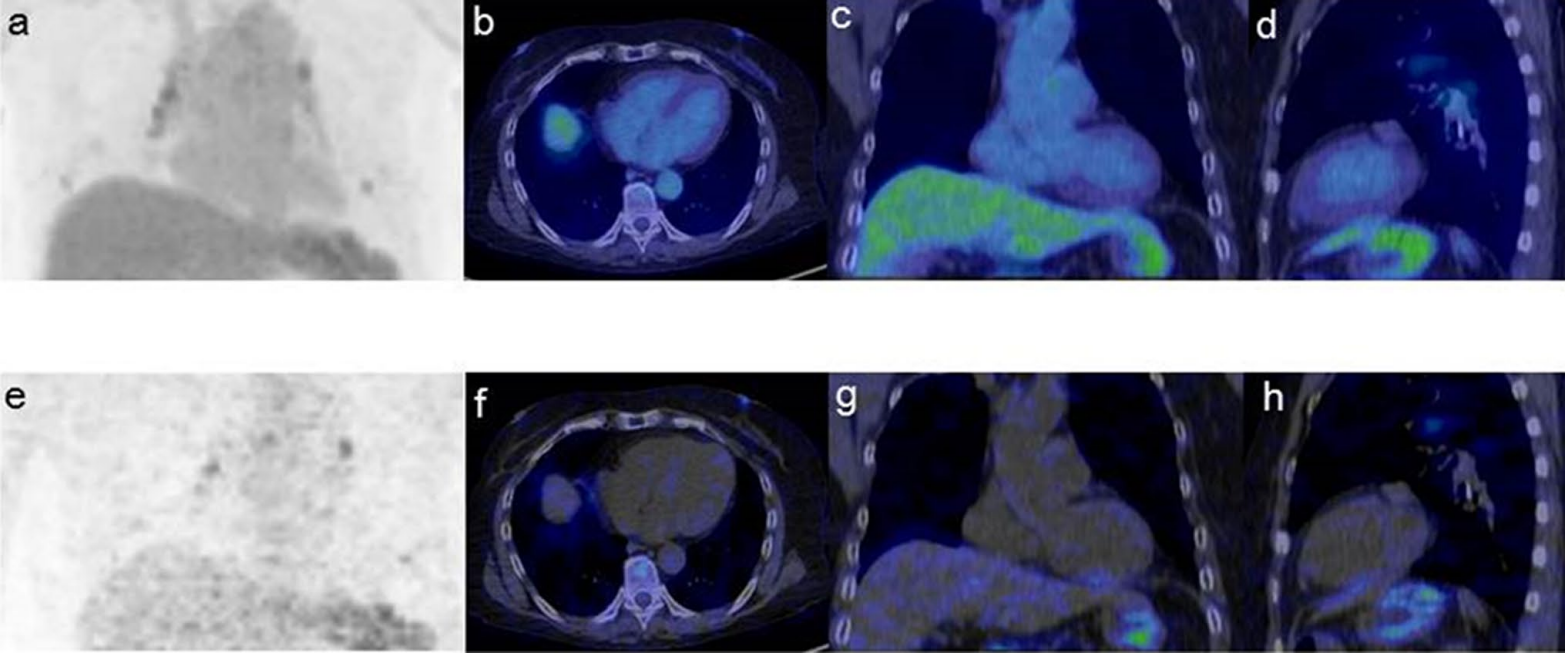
A 69-year-old female patient with CS who showed no arrhythmic events with normal cardiac function (EF: 70%) under steroid treatment (prednisolone, 10 mg daily). The SUV images [MIP (a), transaxial (b), coronal (c), and sagittal (d)] and Ki images [MIP (e), transaxial (f), coronal (g), and sagittal (h)] both show the negative myocardium.
